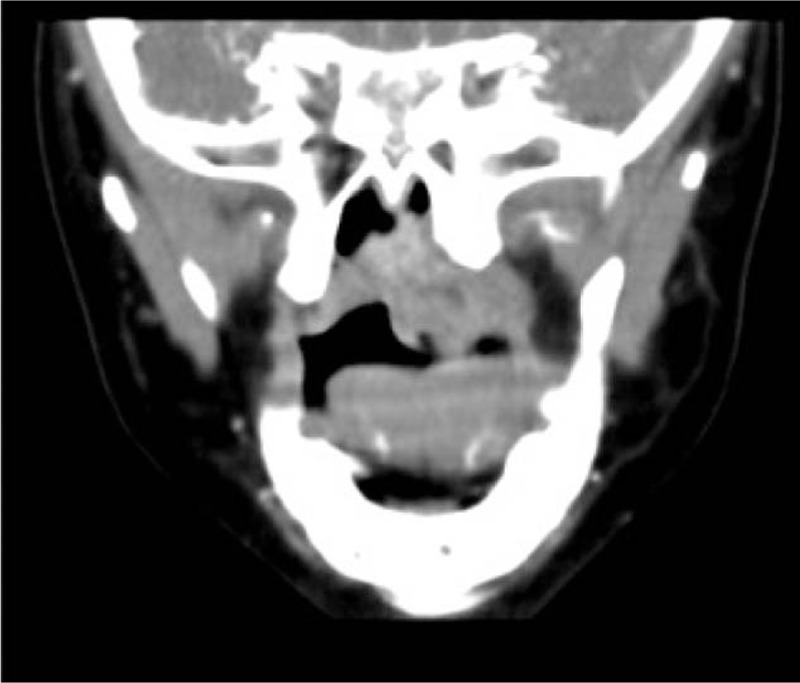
Coronal CT image showed a mass convex to the pharyngeal cavity (about 27 mm × 21 mm × 26 mm in size). CT = computed tomography.
